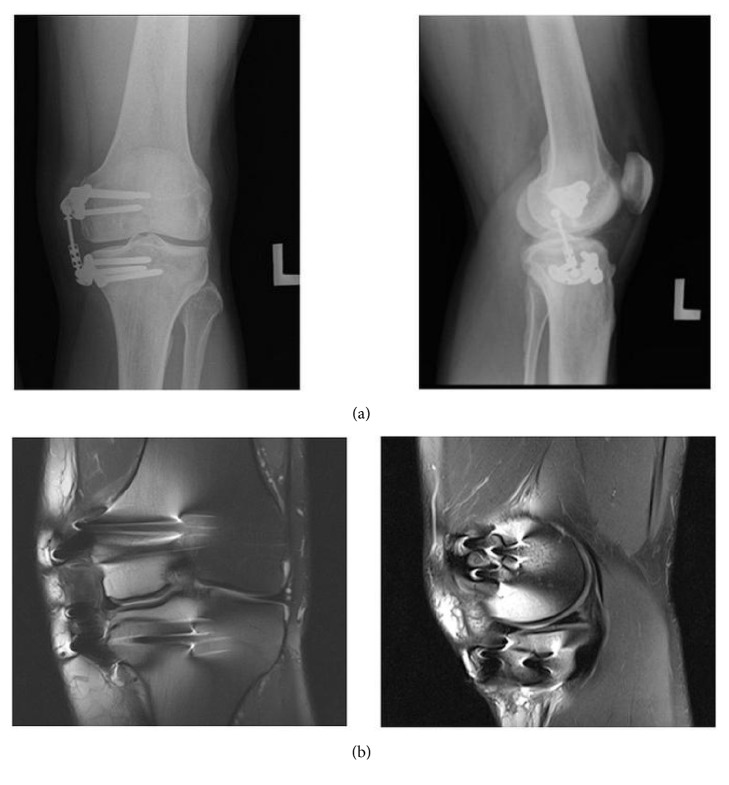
Six-month postsurgery anterior and medial radiographs (a) and coronal and sagittal MRIs (b) of the affected left knee.
